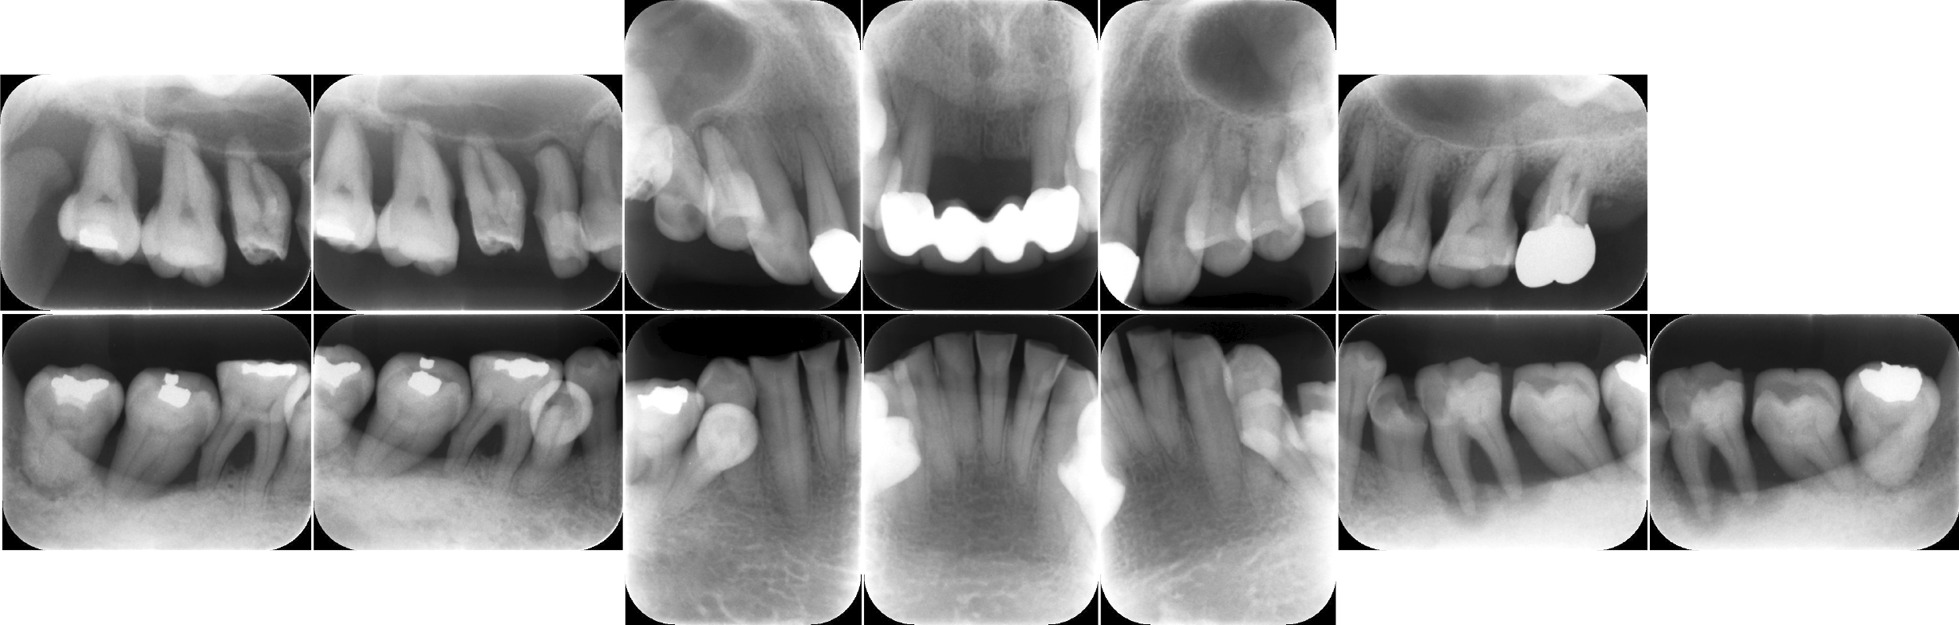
Initial periapical radiographs. Radiographs reveal widespread horizontal bone loss extending to the root apex
